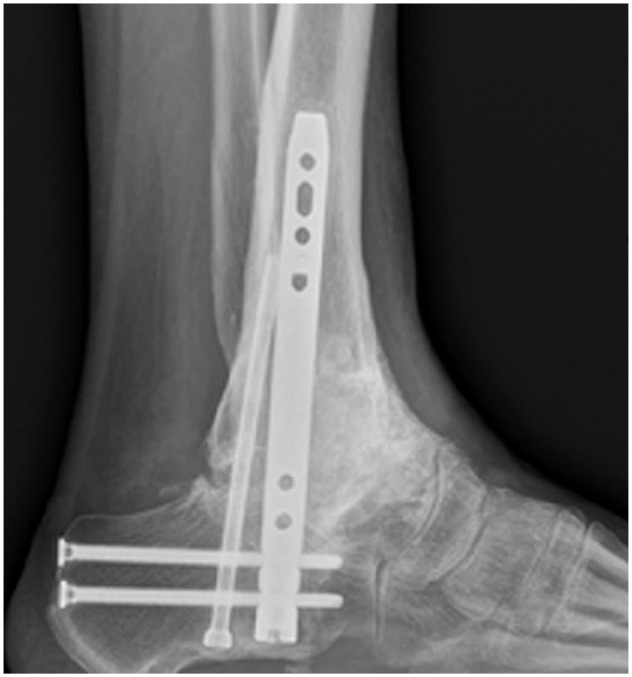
Postoperative lateral radiographs of the case from Figures 1 and 4 showing the extent of solid consolidation of all bone graft. The extra screw in the anterior talus had been removed alongside shaving down of anterior bone.
